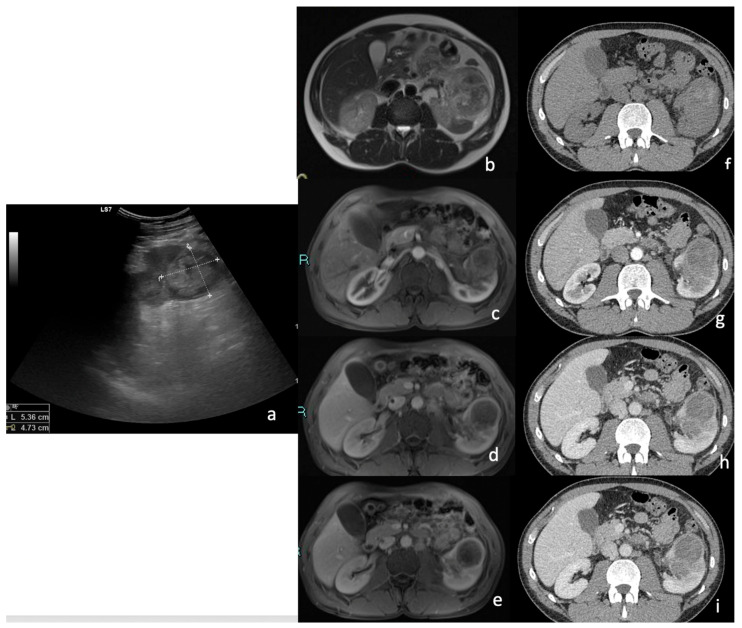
(a) B-mode ultrasound showed an inhomogeneous hyperechoic exophytic renal lesion. The axial T2-weighted image (b) shows a left cortical, exophytic, interpolar renal mass that was inhomogeneous and moderately hypointense in the T2-weighted image; in the axial post-contrast images, in the (c) arteriosus phase (d), venous phase, and (e) delay phase, the lesion was homogenously enhanced and there was no significant washout in the delayed phase. Axial unenhanced (f) and arteriosus-, venous-, and delay-phase (g–i) CT images showed a complex mass in left kidney with intralesional high-density foci in non-contrast images. After contrast, the lesion did not show significant enhancement.
